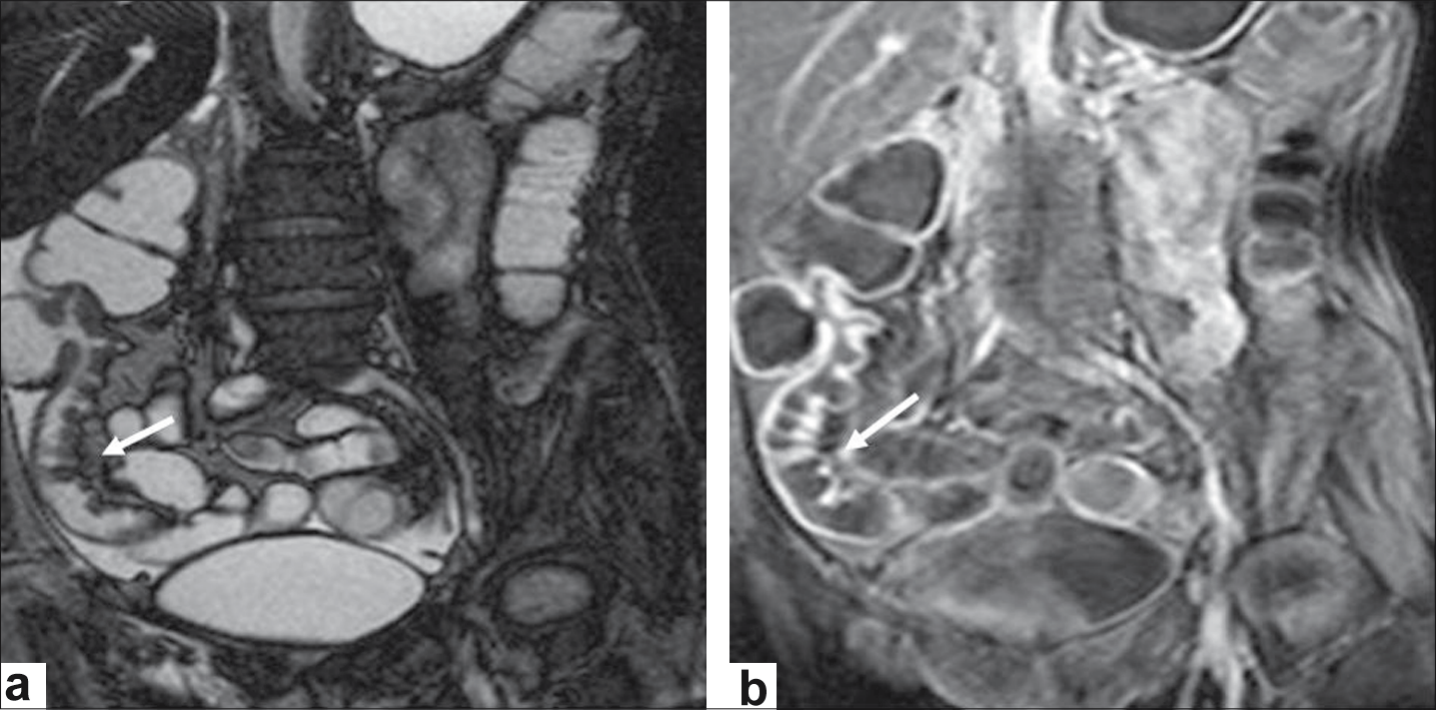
A 40 year-old female patient with persistent diarrhea, crampy abdominal pain, fever, and rectal bleeding. (a) Coronal balanced GRE and (b) coronal FFE T1-weighted image after intravenous gadolinium administration demonstrate the irregularity of the terminal ileum with contrast enhancement (arrows)
